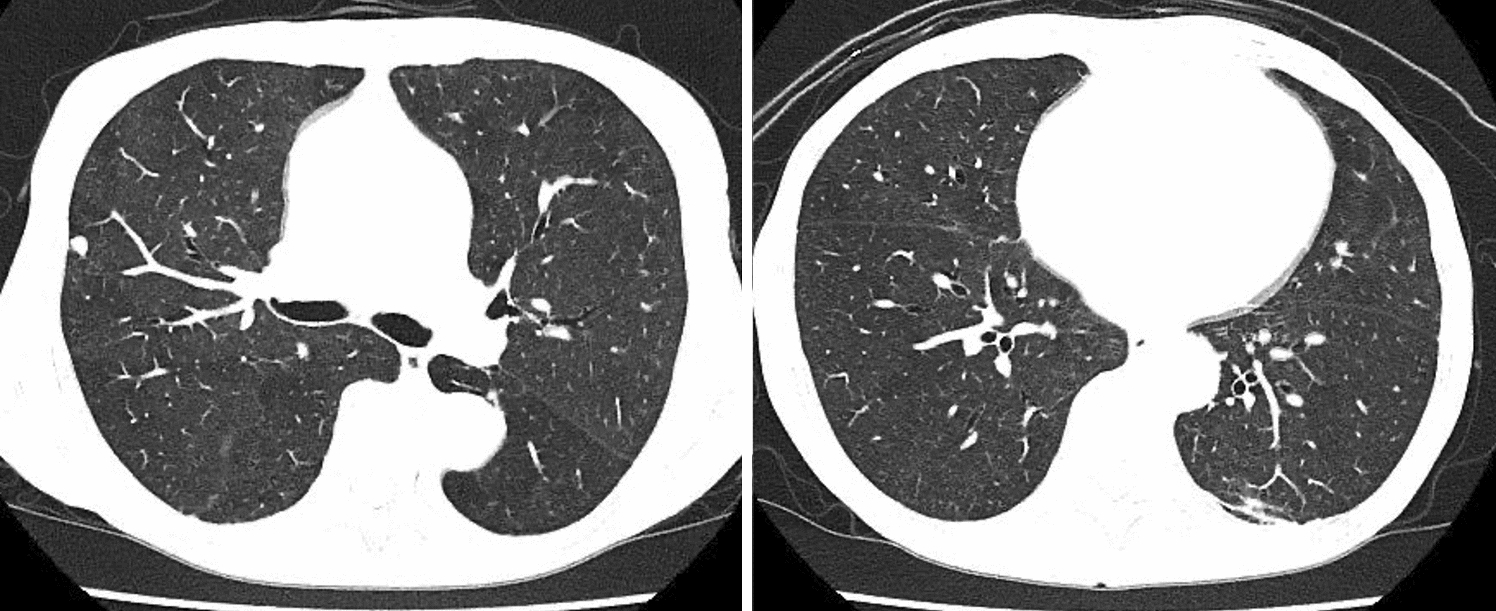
Chest computed tomography scan shows small ground-glass opacities in the bilateral lung fields
